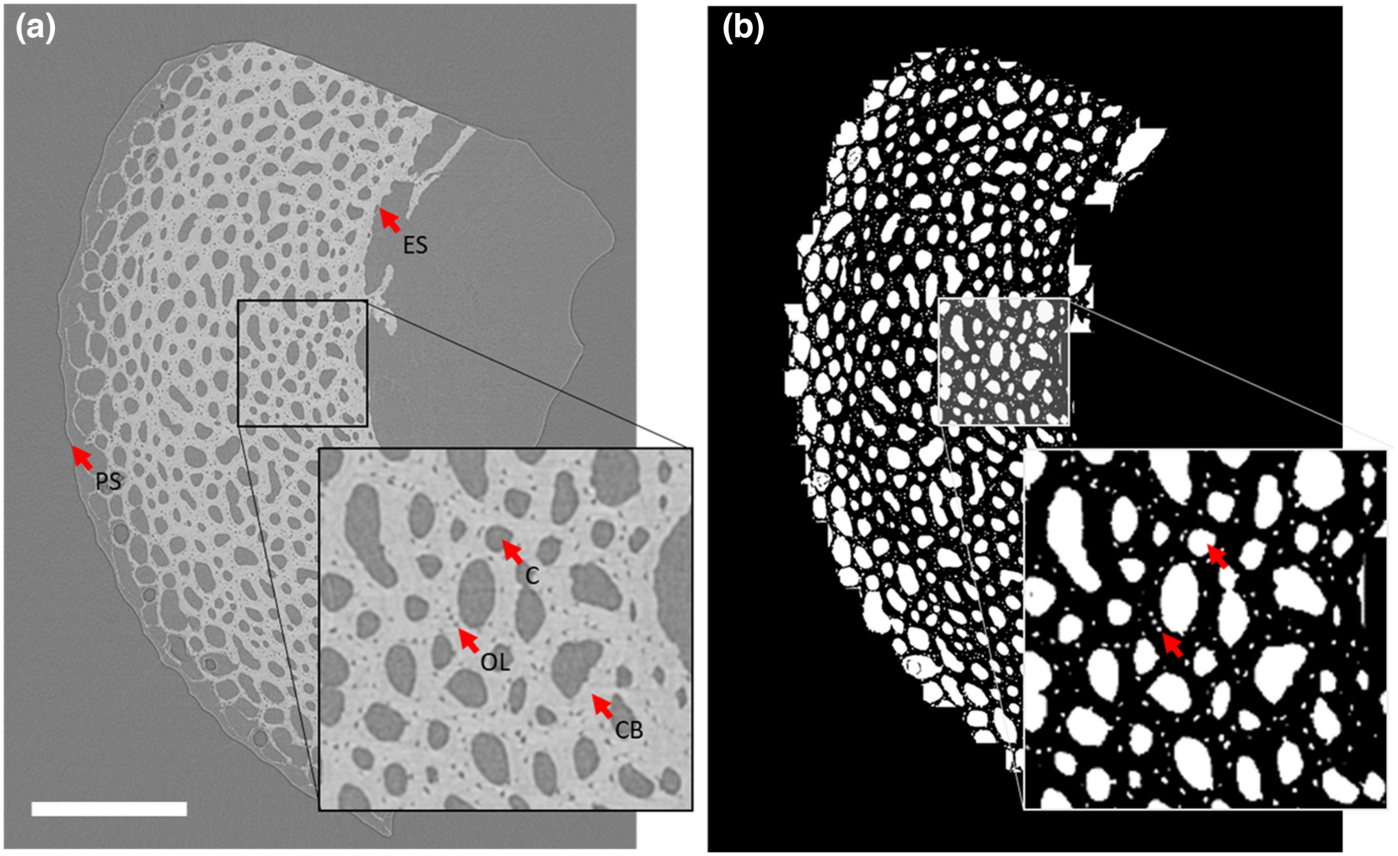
Pre‐ and post‐segmentation images for juvenile duck bone (age 15 days). (a) Greyscale slice from SR CT stack. (b) Segmented pores showing both vascular canals and osteocyte lacunae. PS, periosteal surface; ES, endosteal surface; OL, osteocyte lacuna; CB, cortical bone; C, canal. Scale bar = 500 μm.
